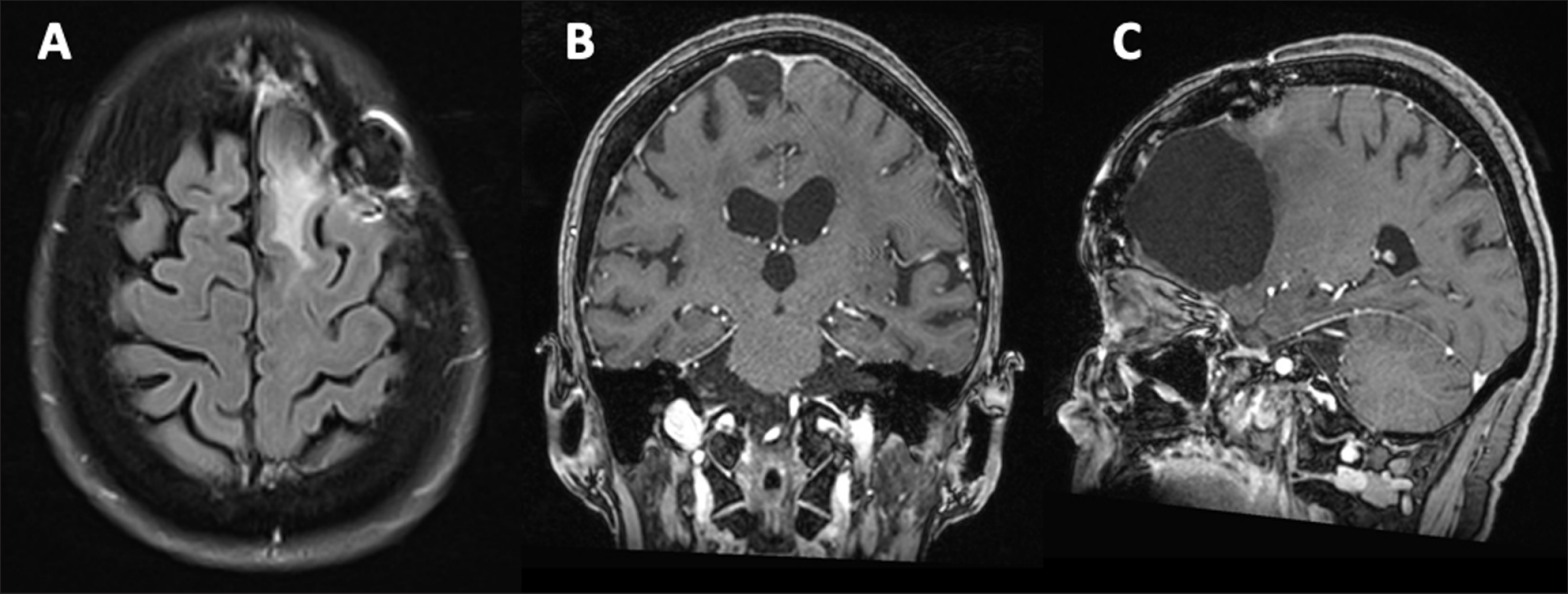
Complete resolution of findings one month later on axial FLAIR (A), coronal and sagittal postcontrast T1-weighted imaging (B – C).
